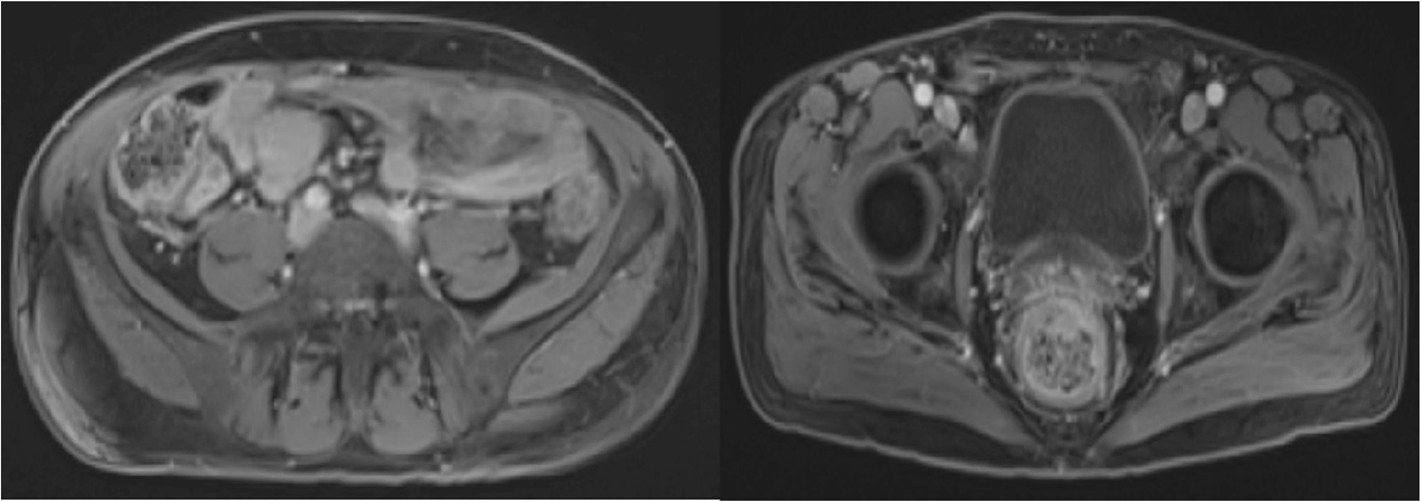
Magnetic Resonance Imaging scan showing near resolution of the circumferential thickening of cecum and rectum after 1 year of antifungal therapy.
